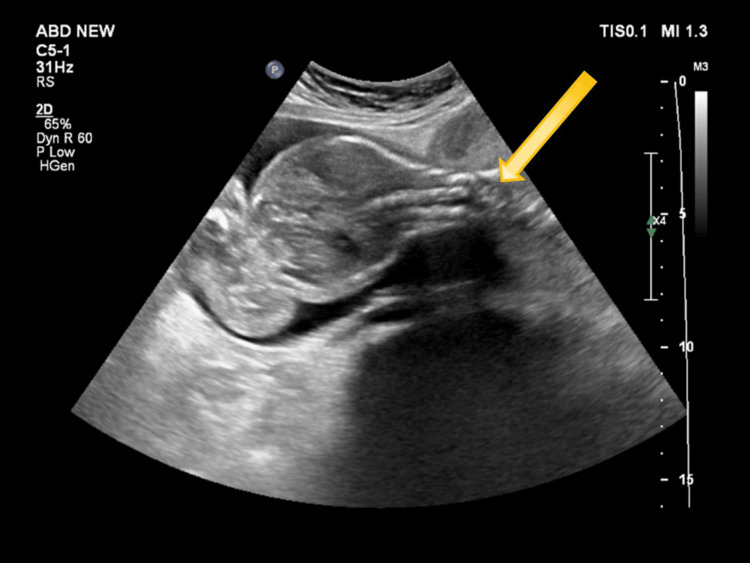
Grayscale ultrasonography image showing a discontinuity in the anterior uterine wall (yellow arrow).
